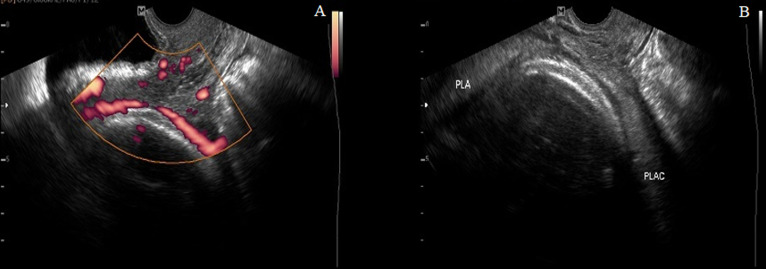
two images stacked side by side showing an interconnecting vessel overlying the internal cervical os on power Doppler (A) and low lying bilobed placenta (B)
